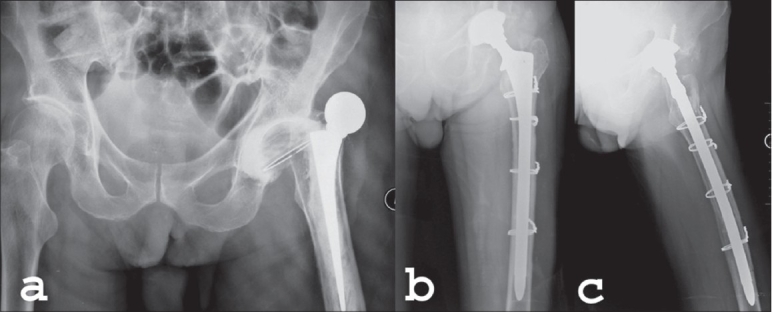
(a) Preoperative X-Ray (AP view) of the pelvis shows three-week-old dislocated retroverted cemented total hip arthroplasty (THA). (b) Postoperative X-Ray (AP and lateral) of left hip at 11 months of cementless revision stem (‘Solution’™) for the failed THA
